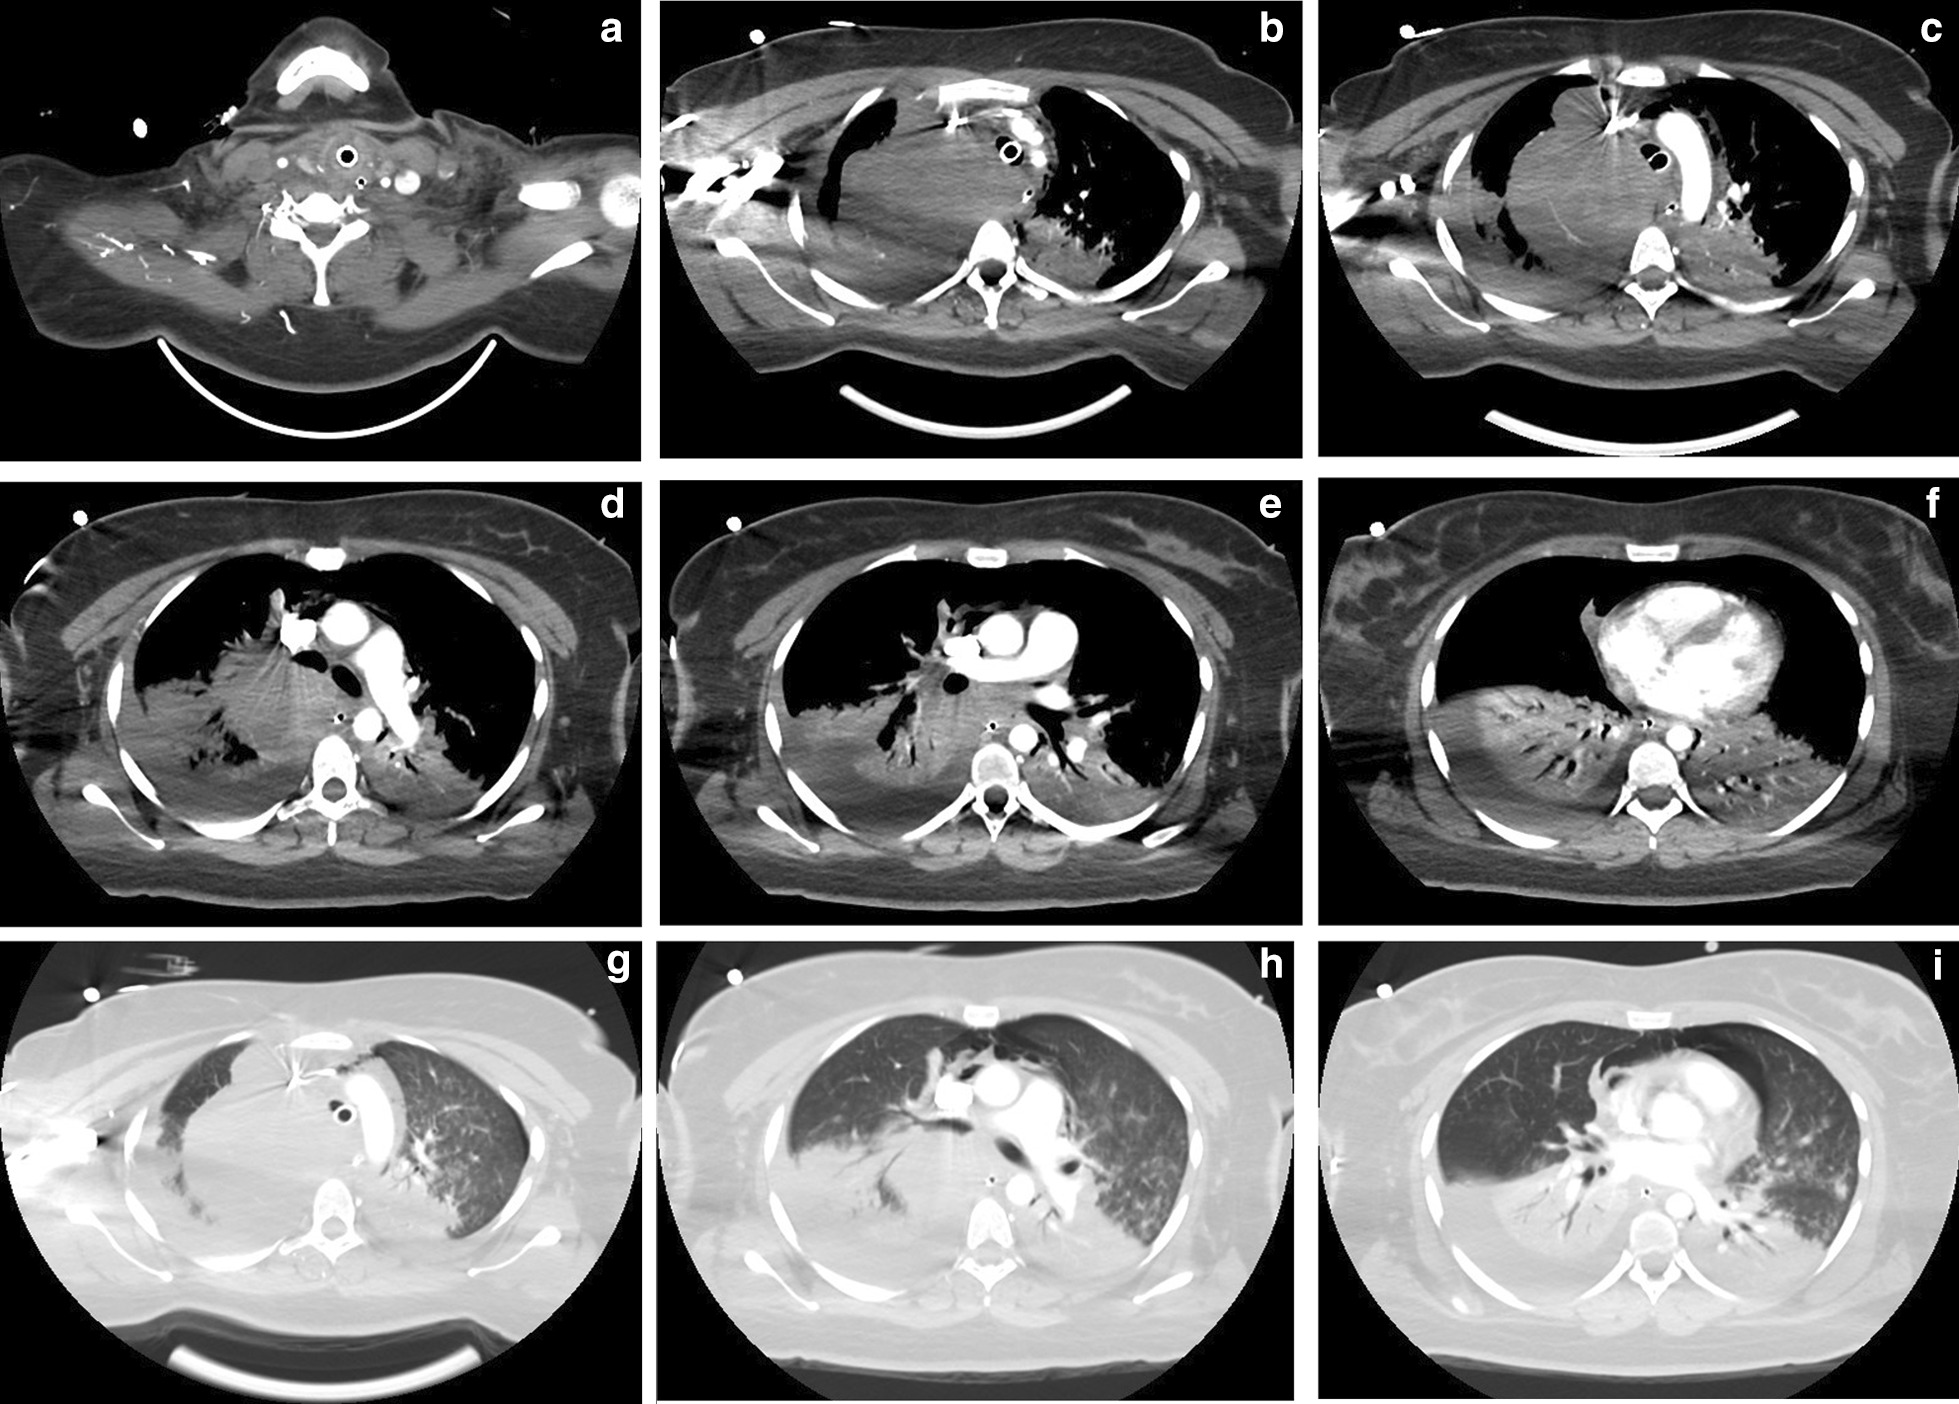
Chest computed tomography scan. a–c Sequence of images of the mediastinal window showing a lobulated, homogeneous mass with well-defined borders that originates from the right thoracic operculum, compressing and displacing the trachea and vessels, with infiltrate surrounding it. d–f Sequence in which bilateral pleural effusion, predominantly right sided, and bilateral infiltrate in the right lower lobes is observed. g–i Lung window displaying the mass, air bronchogram in both lower lobes, pneumomediastinum and bilateral pleural effusion
